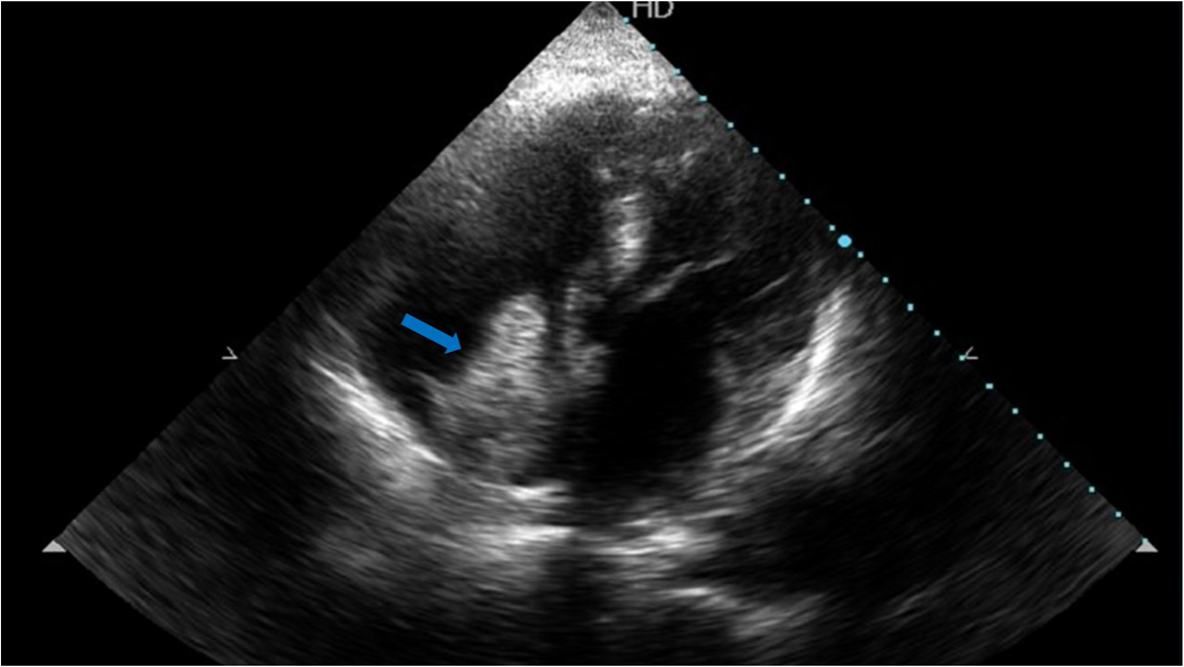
TTE apical four-chamber view showing a large mass in the right atrium marked by a blue arrow
